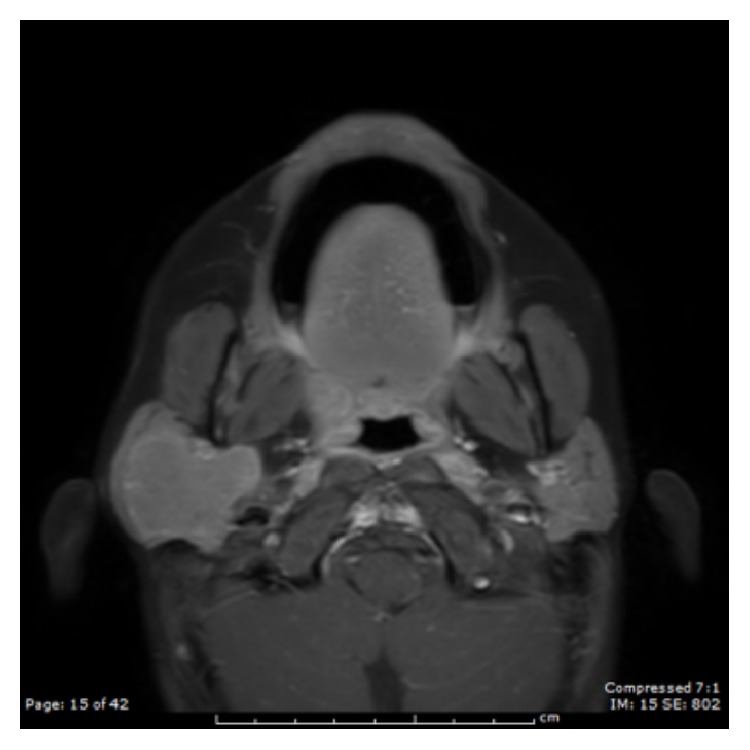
Axial T1 weight MRI (after contrast) shows a homogeneous isointense lesion.
